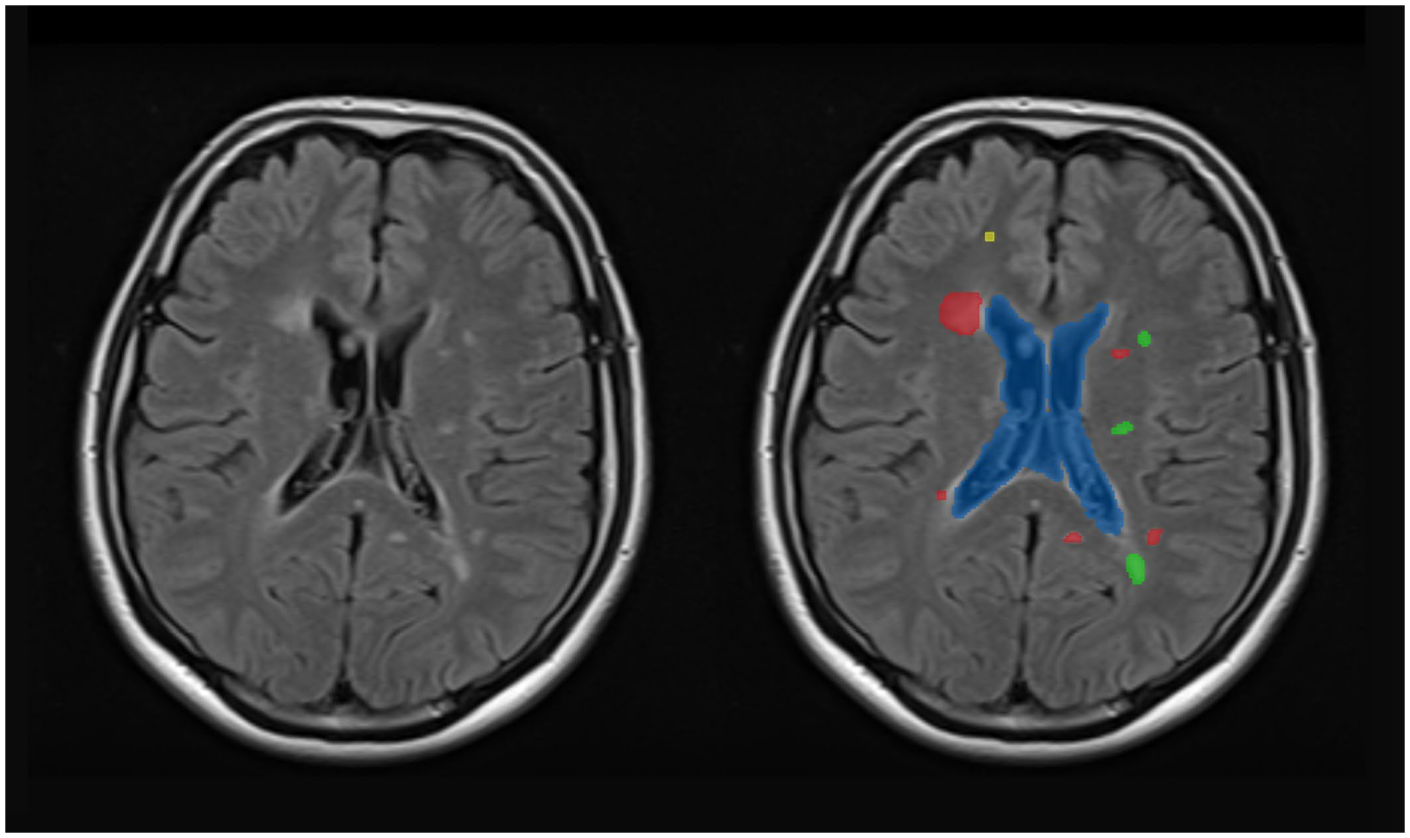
A sample slice of FLAIR images (left) and manually segmented WMH and ventricular system (right). The ventricular system is highlighted as blue, whereas the periventricular, paraventricular, and juxtacortical WMH are differentiated by red, green, and yellow colors, respectively.
